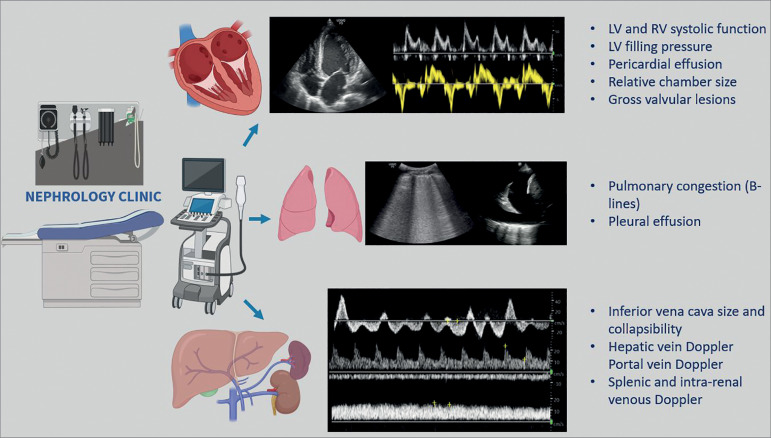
Various components of sonographic hemodynamic assessment performed in the outpatient cardiorenal clinic. Commonly posed focused clinical questions are listed. Representative sonographic images include - mitral inflow Doppler and mitral annular tissue Doppler (cardiac); B-lines and pleural effusion (pulmonary); Doppler waveforms of the hepatic, intra-renal and portal veins from top to bottom (venous). LV = left ventricle; RV = right ventricle.
